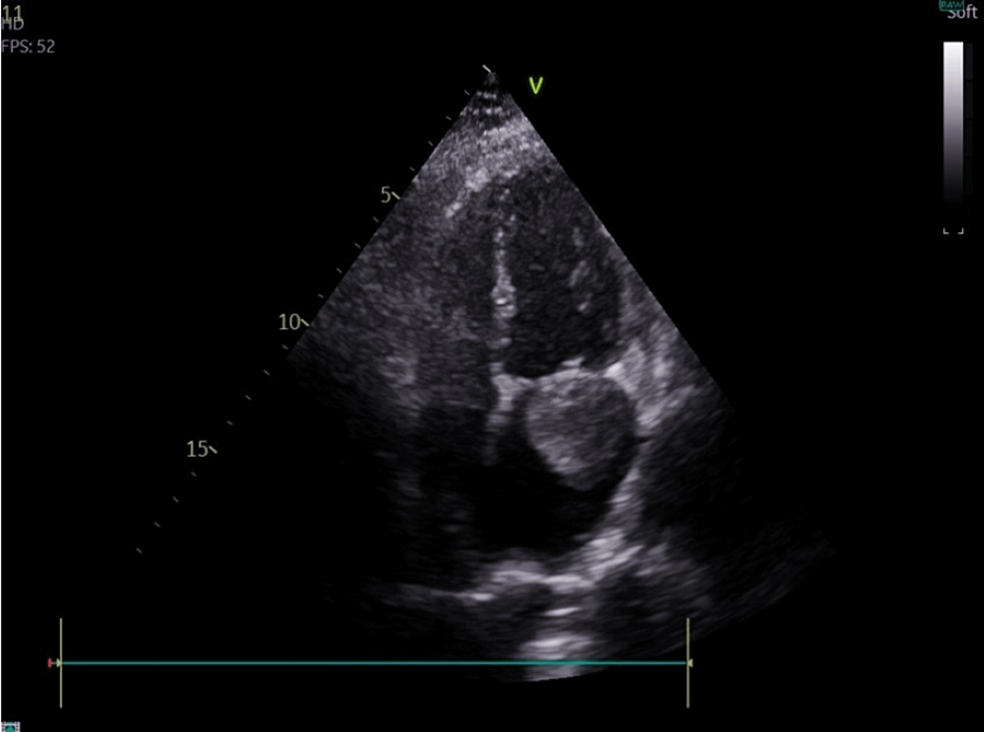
Echocardiographic image obtained in the four-chamber apical view, showing the myxoma in the left atrium
